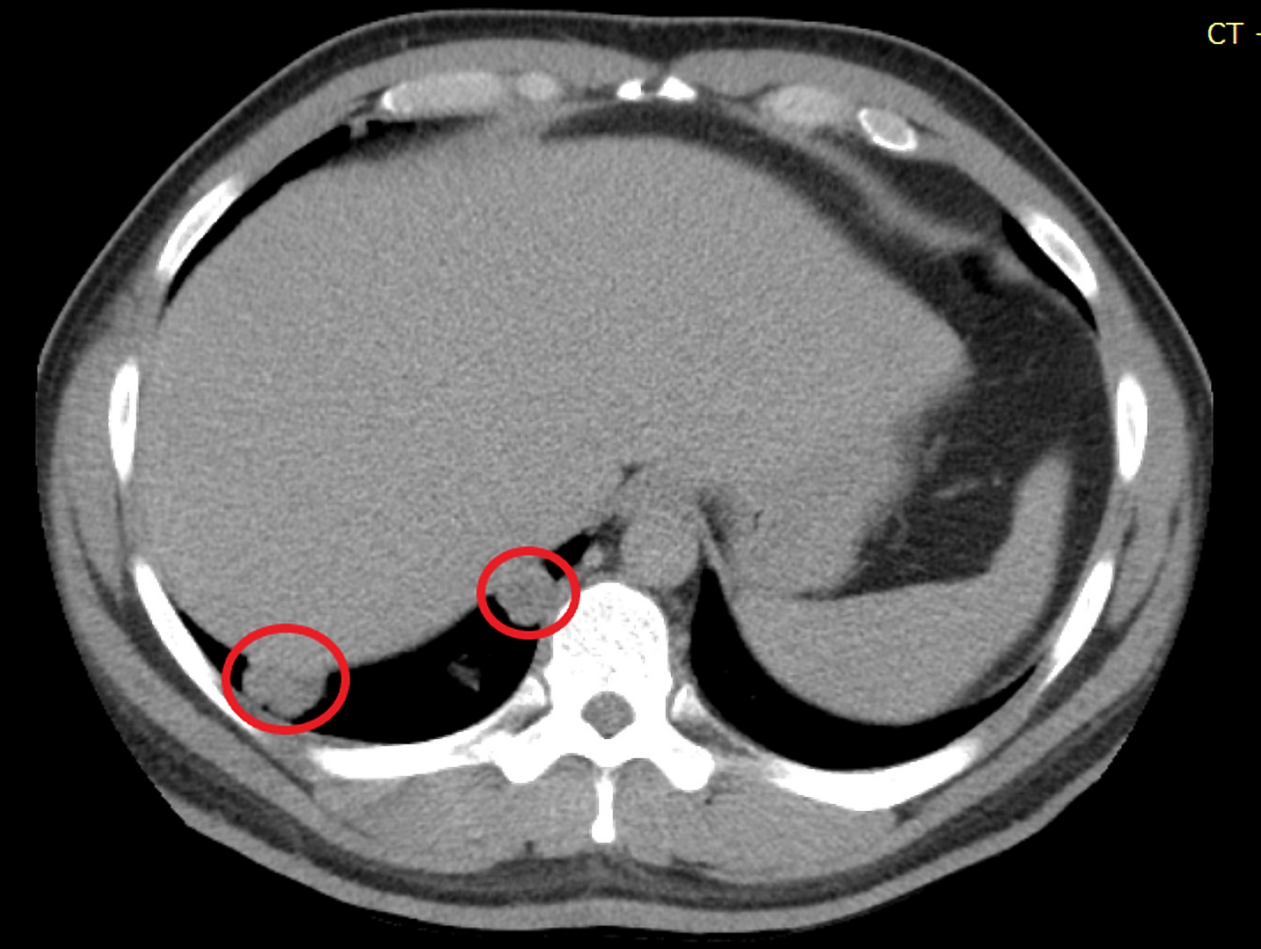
Abdominal computed tomography (CT) scan showing multiple metastatic lesions in the liver (red circles)
